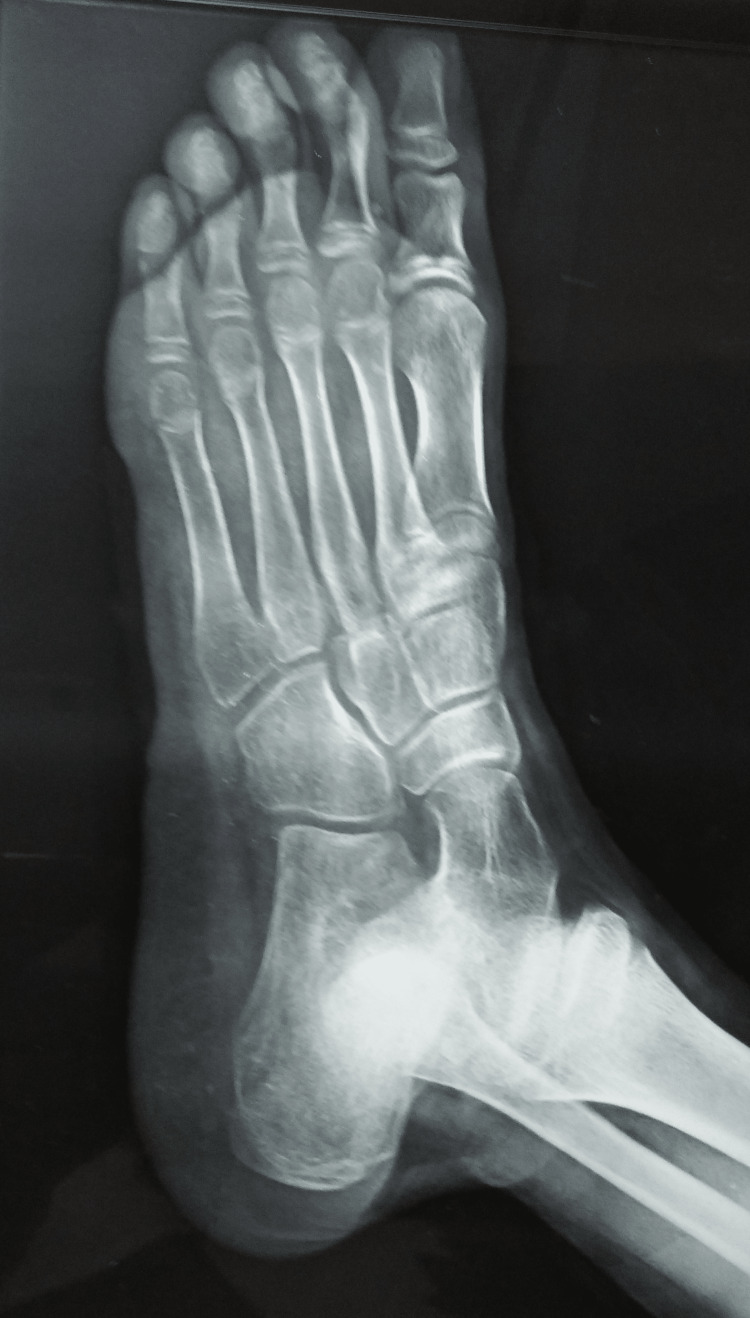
An unremarkable radiograph of the right foot, lateral view
